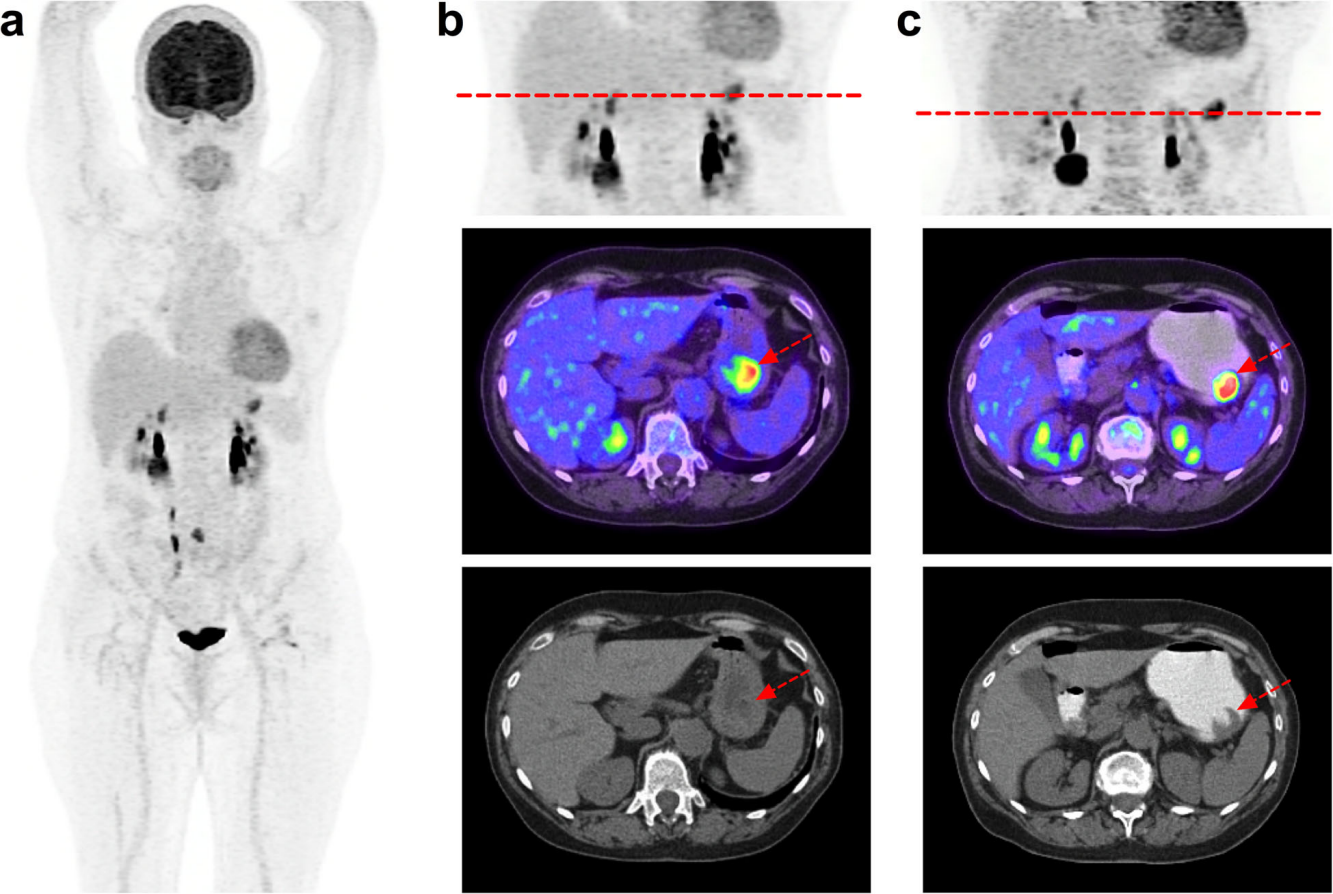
63-year-old woman, referred for assessment of metastatic melanoma (brain, lungs, lymph nodes) after 17 cycles of second-line Pembrolizumab. Her medical record reported anaemia. From left to right, MIP body PET and from top to bottom, abdominal MIP, PET/CT fused and CT axial slices: a and b Images 60 min after FDG injection. c delayed images acquired 15 min after 20 mg scopolamine intravenous infusion and oral contrast agent (500 cc) that permitted to dilate and fulfil the stomach showing an ulcered gastric metastasis, confirmed at endoscopy and likely to be the cause of the reported anaemia
